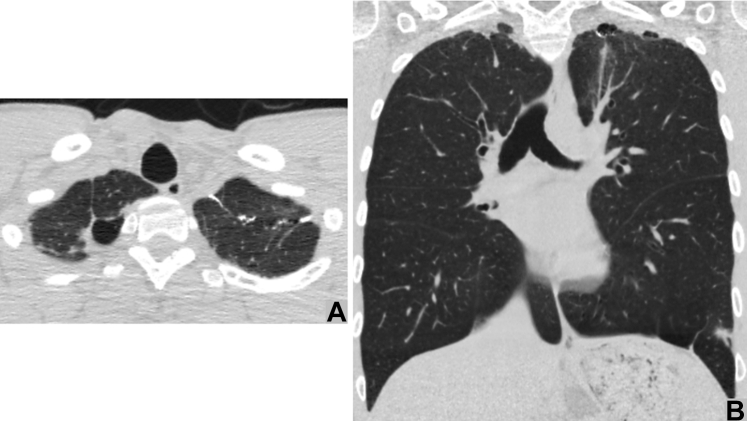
A, B, Chest high-resolution CT scan on referral to our center: (A) axial and (B) coronal reconstructions showing bilateral apical pleural thickening with adjacent mild subpleural reticulation and subtle tractional bronchiectasis, more pronounced on the left side.
